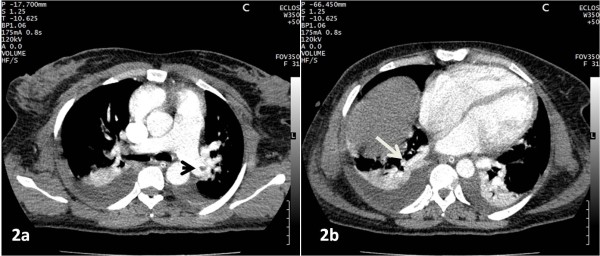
CT angiogram of a 33-year-old woman with clinical suspicion of PE three days after surgery (Caesarean section) shows an embolus in the left pulmonary artery (2a, arrowhead) and in the right postero-basal pulmonary artery (2b, white arrow). Note bilateral pleural effusion and peripheral wedge-shaped opacity.
